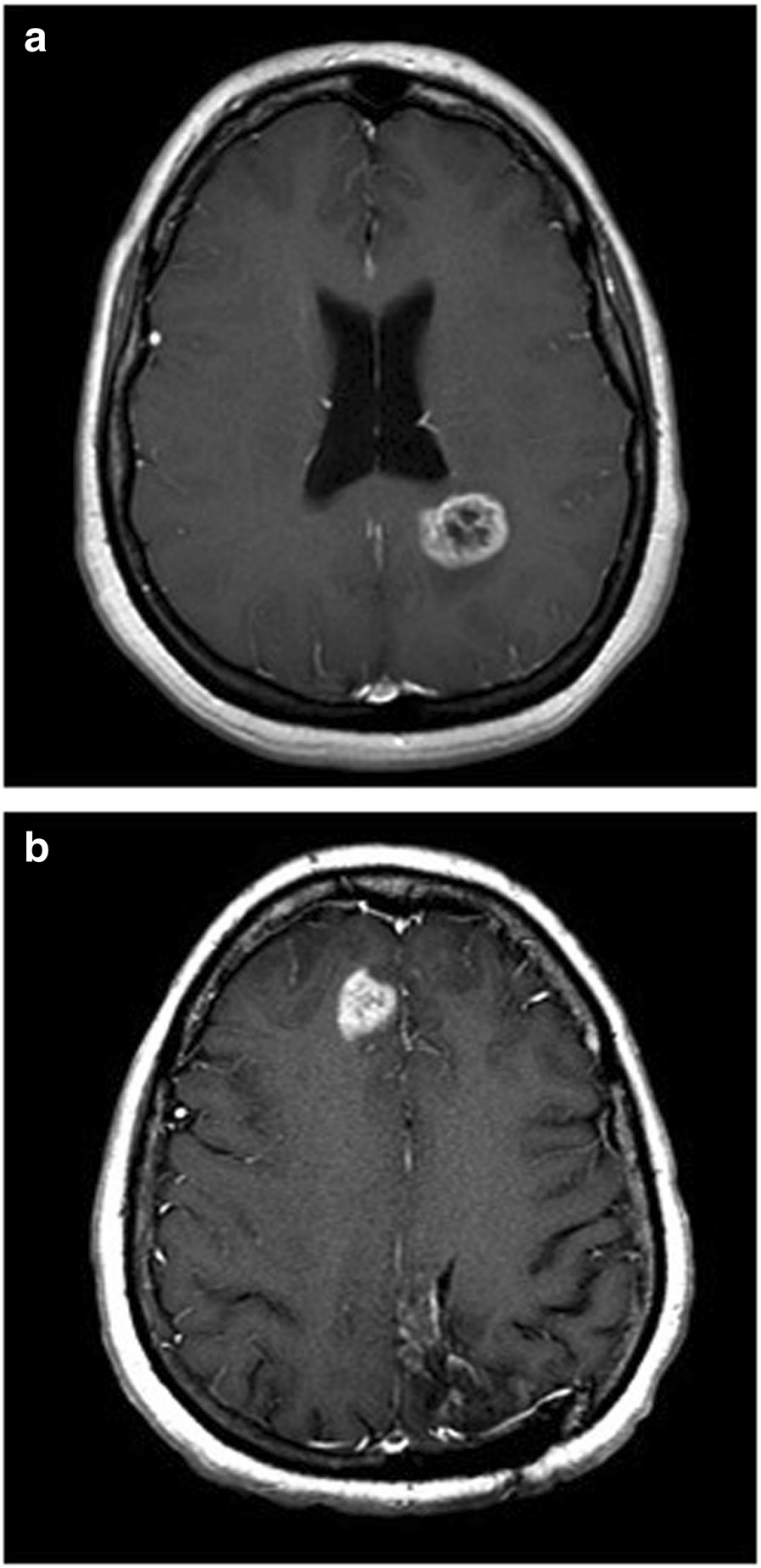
Patient 1 imaging. MRI showing (a) initial left parietal glioblastoma multiforme (GBM) and (b) bifrontal recurrence.
